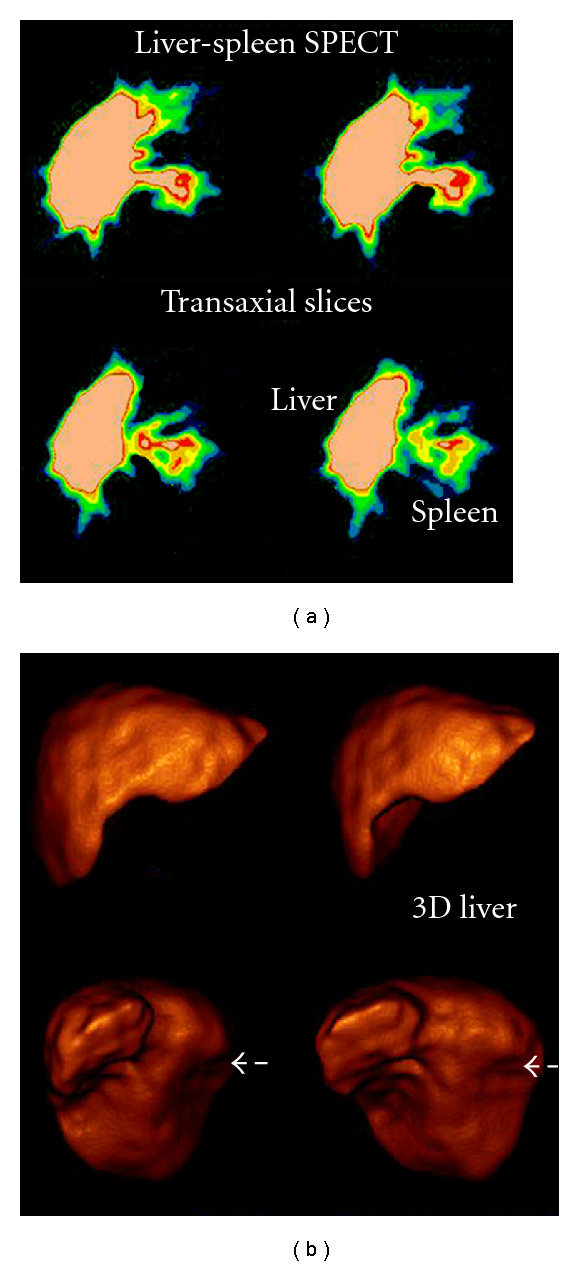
A liver-spleen study SPECT and 3D SPECT. (a) Transverse slices, prefiltered only by Ramp filter could emerge a small piece of the ruptured spleen. (b) 3D SPECT reconstruction by ramp-Hanning filters (Hanning cut-off frequency equal to 0.8 cycles/cm), a threshold 25% and 15% gradient, could show the liver and a short fracture in the middle of right lobe of the liver but misses any residual spleen fragment. (*Study has been offered by Radiation Physics Unit, Department of Radiology, University of Athens)*.
